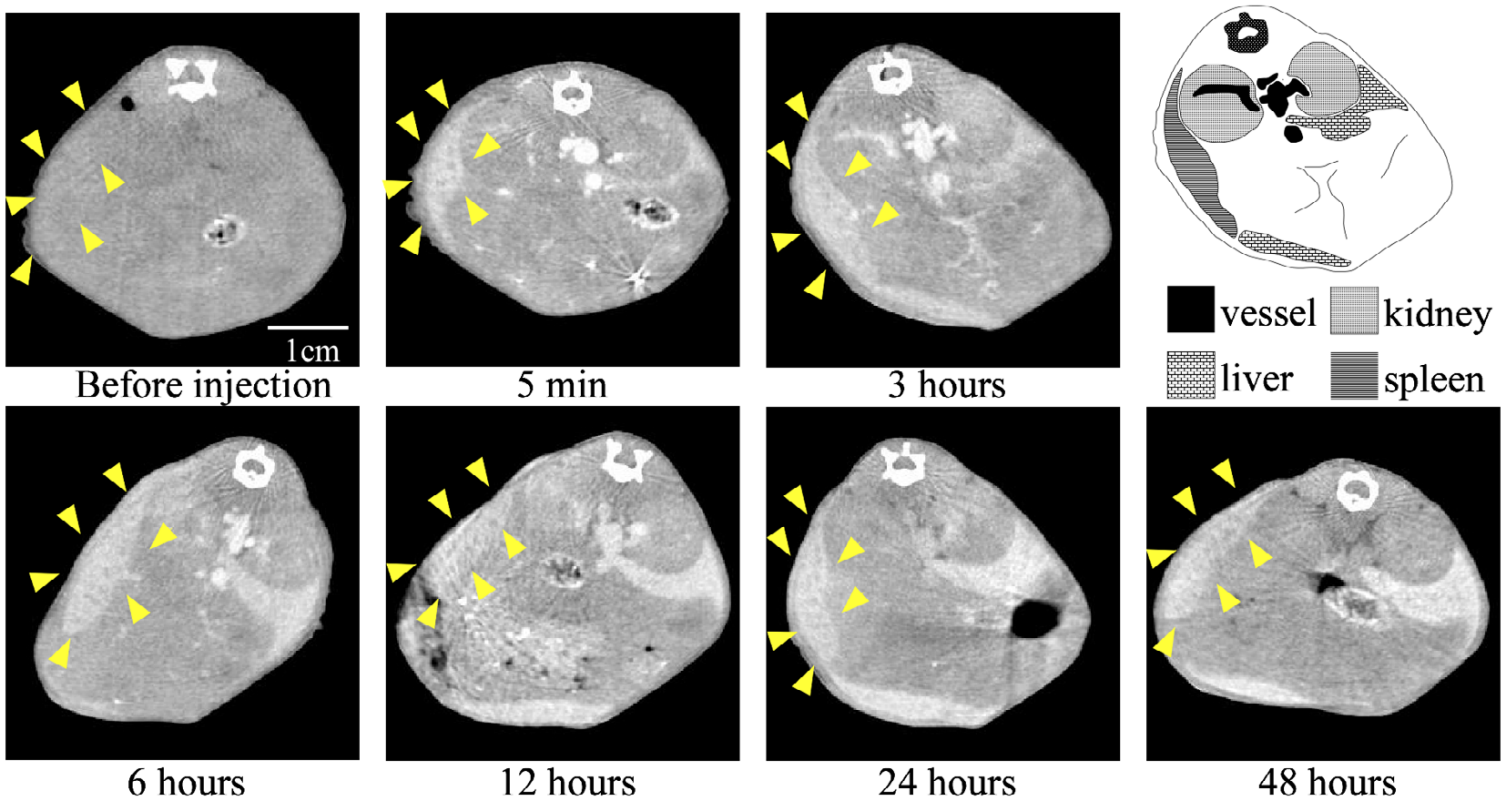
Change in CT value of a spleen slice before and after injection of 30-nm Au-PEG. Yellow arrowheads show the region of the spleen.
